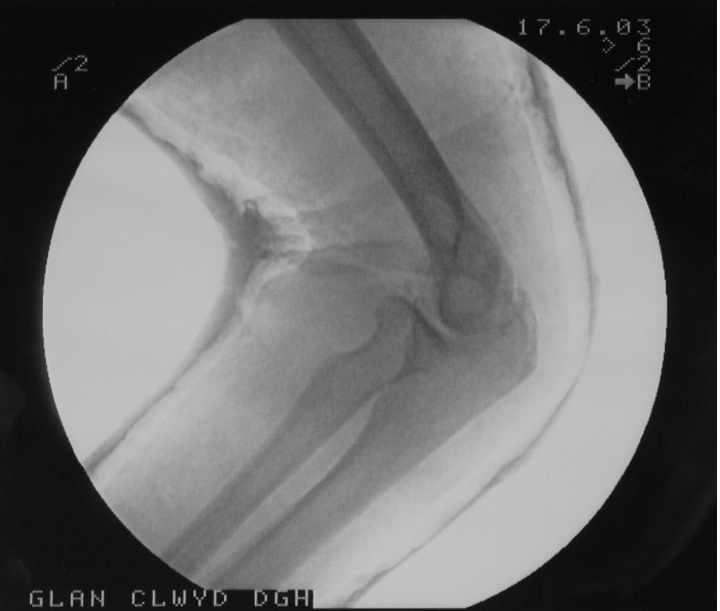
Post reduction lateral radiograph of the elbow showing the radial head in reduced position.
